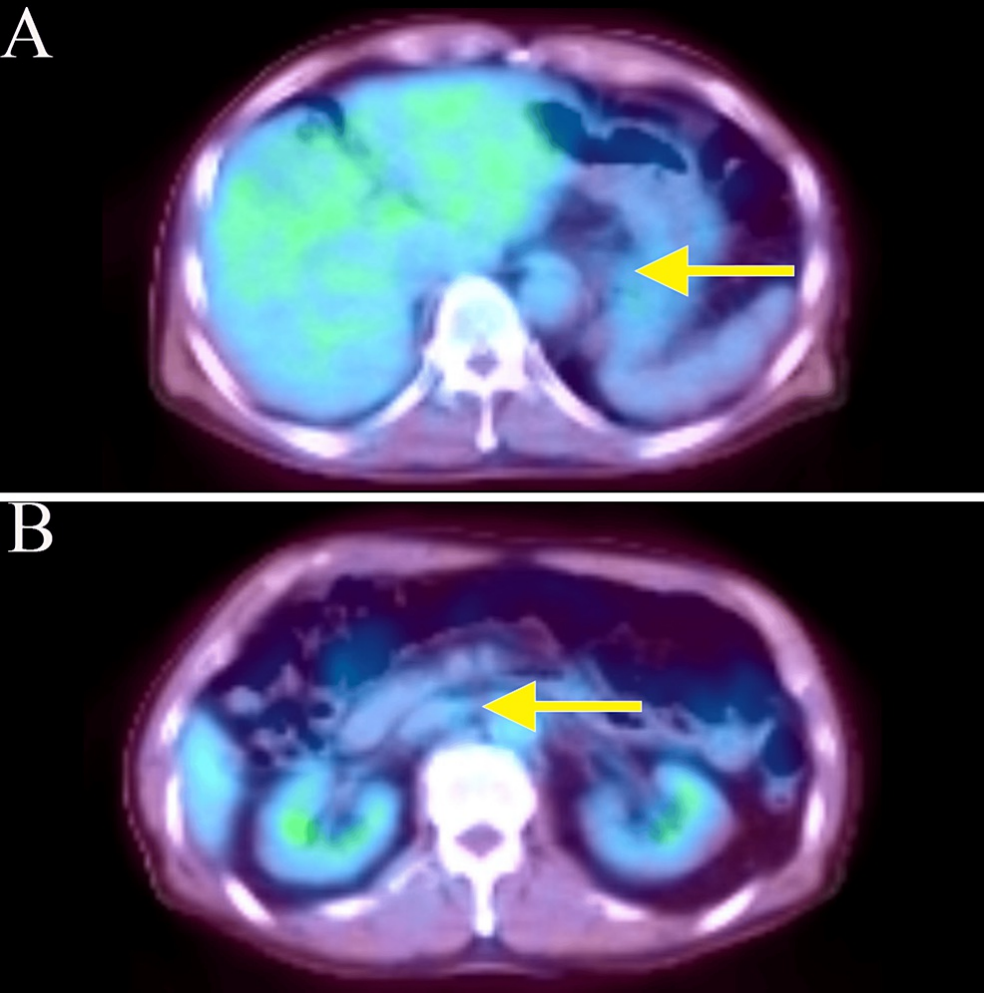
Positron emission tomography-computed tomography (A, B) Images taken after chemotherapy. PET-CT scans showed that the tumor had almost disappeared.
